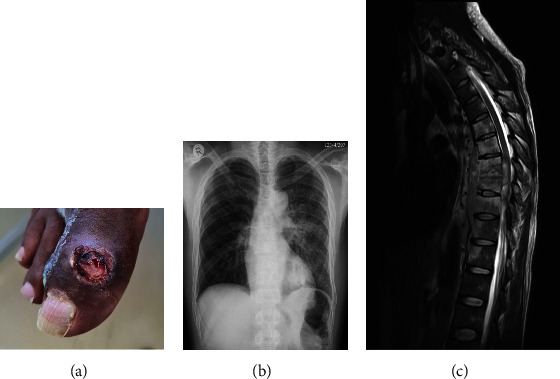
(a) Right hallux non-healing ulcer, (b) chest radiograph, and (c) magnetic resonance imaging (MRI) of the thoracic spine.
